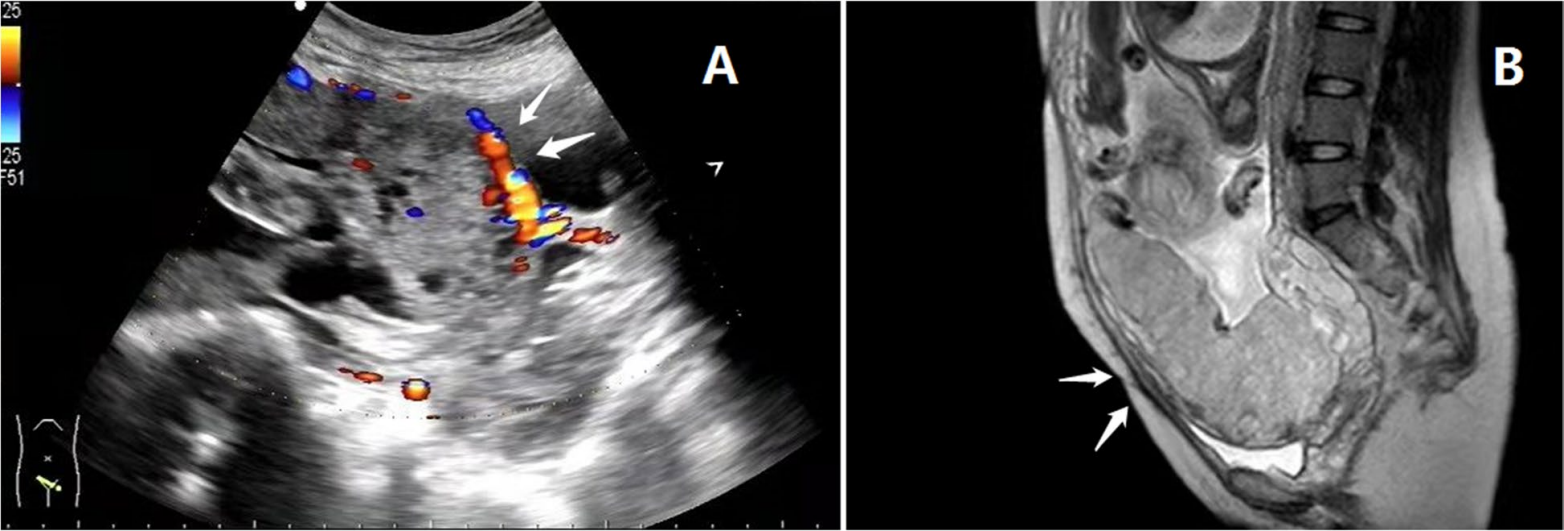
A Color Doppler images. The arrow indicates evidence of placenta accreta (indistinct boundary of utero-placental interface); B MRI. Placenta accreta in the lower uterine segment (indicated by an arrow)
